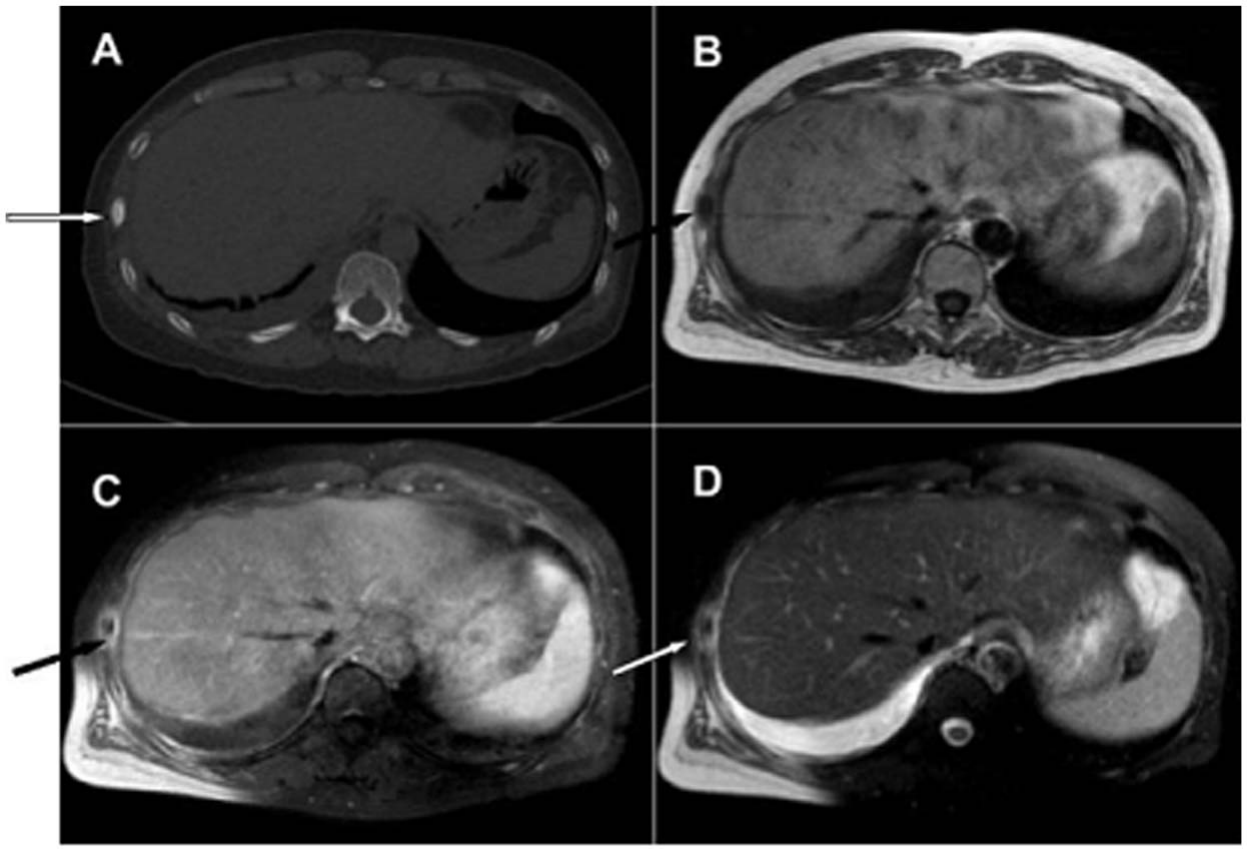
MRI in the detection and extent of osteoblastic metastatic lesions through direct and indirect signs. A. CT image showing an osteoblastic metastatic lesion of a right rib, presenting as an intramedullary homogeneously high density (white arrow). B. T1WI showing an area of low signal intensity (black arrow). C. The lesion enhances slightly and heterogeneously on Gd-DTPA enhanced T1WI imaging with peripheral edema (black arrow). D. T2WI (FS) showed intramedullary areas of high, slightly high, isometric and low-mixed signal intensity with peripheral edema (white arrow).
